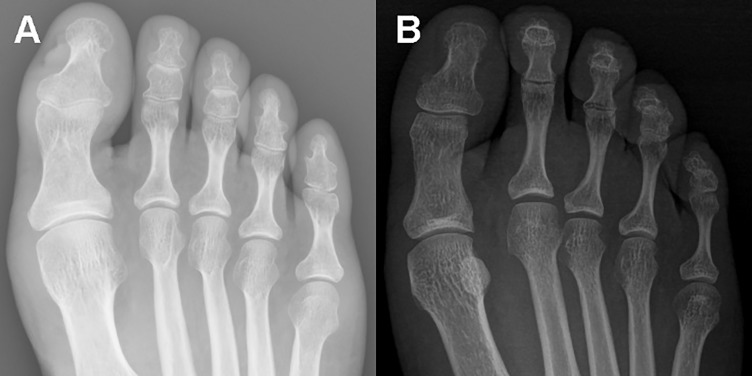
Anteroposterior radiograph of the forefoot in two adults: (A) absence of both hallucal sesamoid bones, which is a rare variation; (B) absence of the medial sesamoid bone at the hallucal metatarsophalangeal joint.
